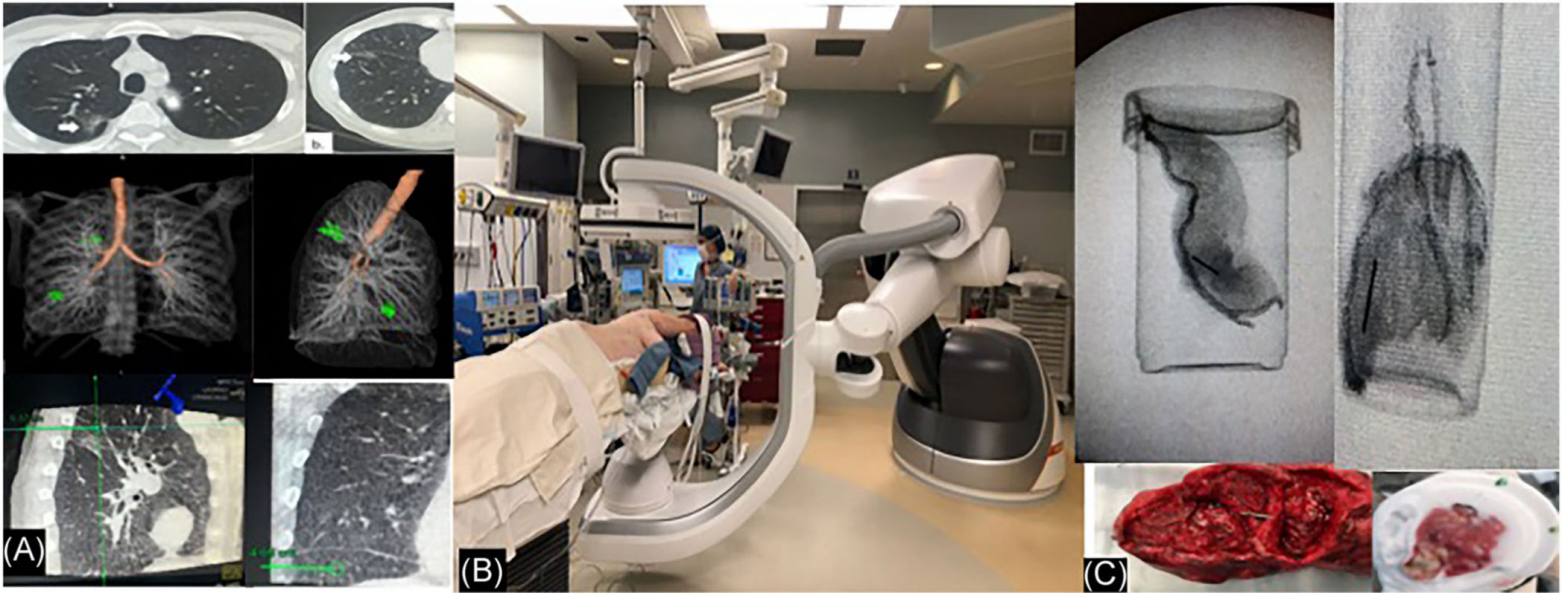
A, Axial computed tomography (CT), 3D volume rendered and sagittal and coronal cone beam CT images, of patient with two nodules resected in the same procedure; the size, morphology location, and T-bar path (green lines) are depicted
